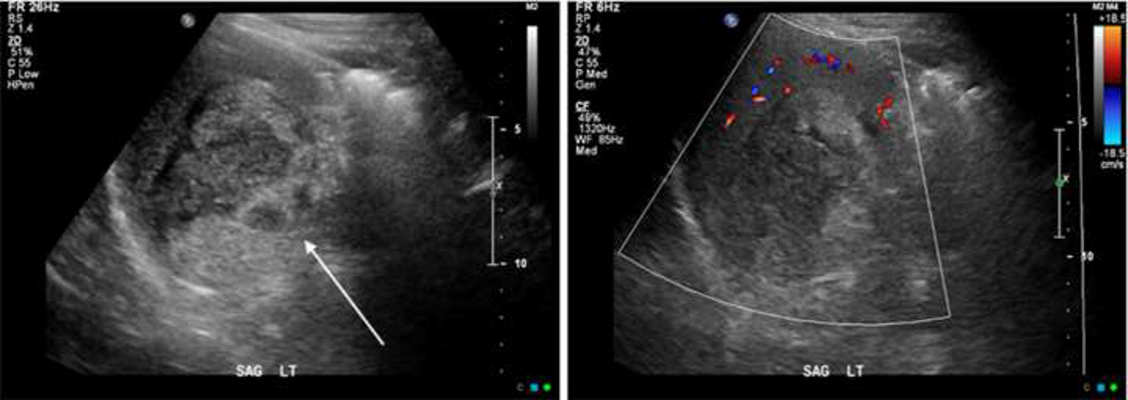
Ultrasound images showed a hypoechoic splenic mass with no internal vascularity (arrow). There were no focal lesions in the liver.
